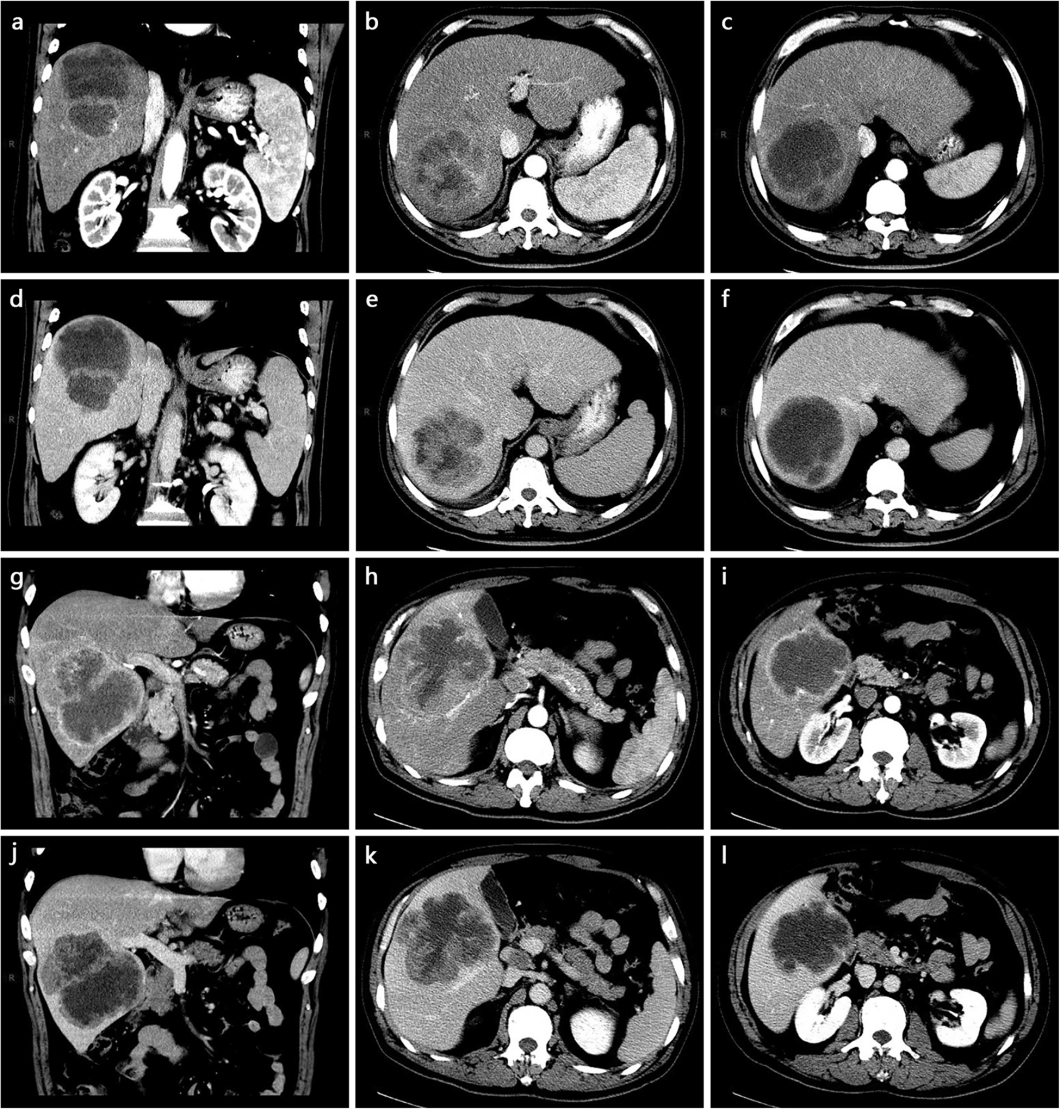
Hepatic leiomyosarcoma in a 62-year old man (a-f) and sarcomatous hepatocellular carcinoma in a 56-year old man (g-l). On the contrast-enhanced dynamic CT coronal and axial images, hepatic leiomyosarcoma (a-c) exhibits isoenhancement on the AP and persistent or progressive enhancement into the center on the later phase (d-f). The sarcomatous hepatocellular carcinoma presents obvious peripheral enhancement on the AP (g-i) and subsequent wash out (j-l)
